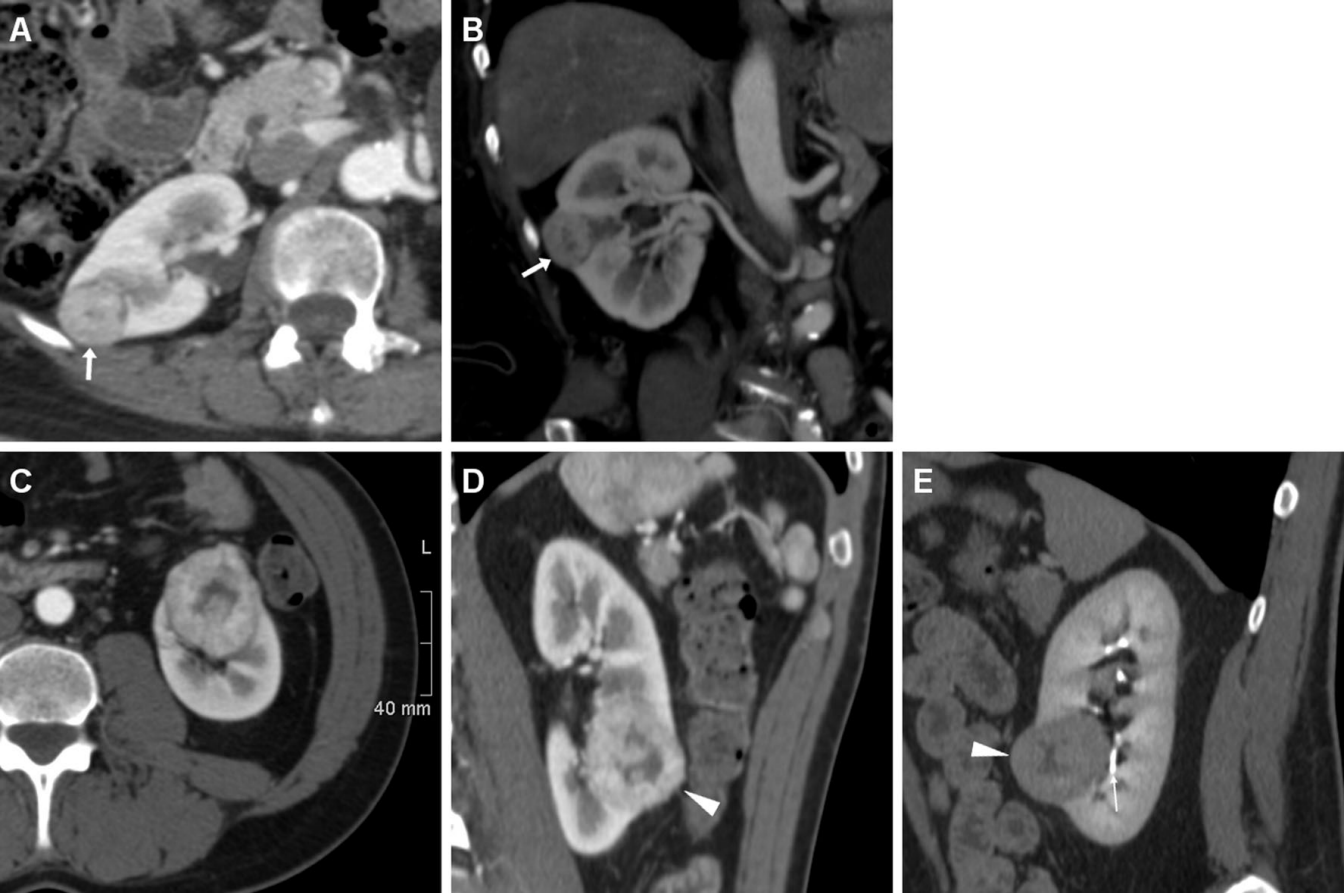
Examples of RENAL nephrometry scores. Total body contrast-enhanced multidetector CT (a, b) performed for clinical suspicion of systemic lymphoproliferative disease in a healthy 61-year-old woman led to incidental detection of a 2.5-cm, vascularised, partially (<50 %) exophytic mass at the middle third of the right kidney (*arrows*), consistent with T1 stage renal cell carcinoma (RCC). Nephrometry resulted 1 + 2 + 2 + p + 3 (8p). The patient underwent laparoscopic nephron-sparing surgery (L-NSS) complicated by haematoma (*see* Fig. 5). Multiphase CT-urography (c–e) in a 55-year-old man confirmed ultrasound detection of a 43-mm, inhomogeneously enhancing, left-sided RCC. The lesion appeared partially exophytic, crossed the inferior polar line (d) and touched the lower pole calyx (e). Nephrometry score was 2 + 2 + 3 + a + 2 (9a). The patient successfully underwent laparoscopic partial nephrectomy (L-PN)
